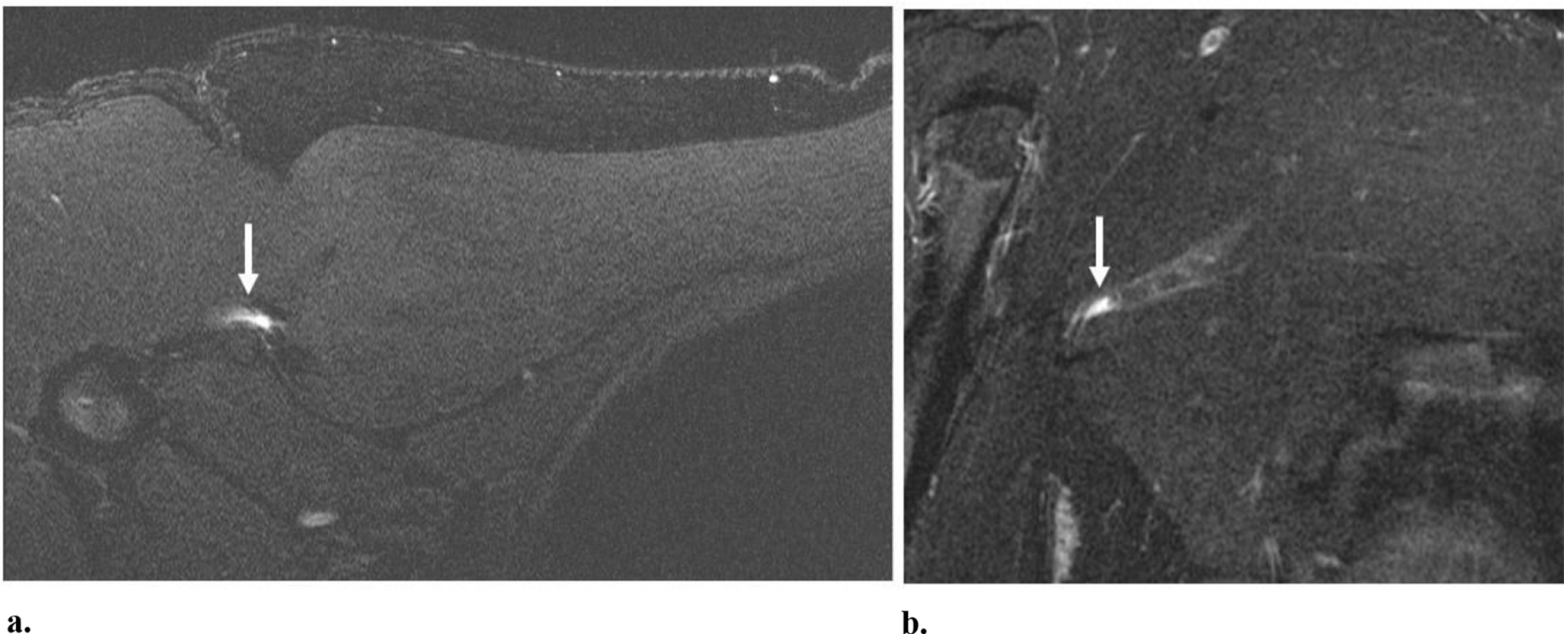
18-year-old-male that presented after pain with weightlifting. (a) Axial T2-weighted-fat-saturated and (b) coronal oblique STIR- weighted MR images show edema-like signal along the myotendinous junction (arrow) of the sternal head of the pectoralis major, representing partial-width tear
